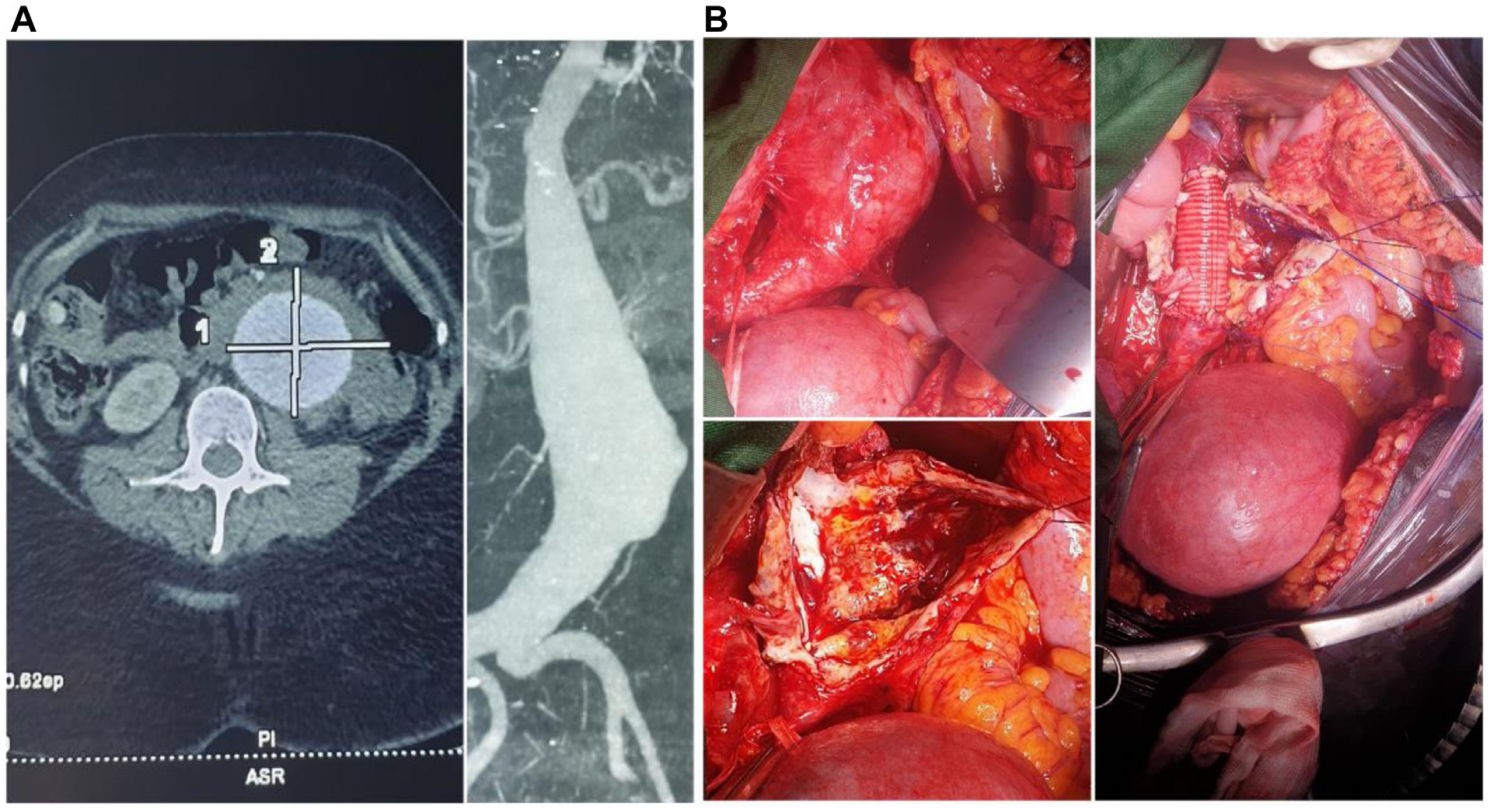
(A) Axial view of angio-computed tomography (CT) scan showing a large abdominal aortic aneurysm (AAA) (left) and two-dimensional MPR reconstruction (right). (B) Intraoperative view of the AAA (upper left corner) before “mise-à-plat” (lower left corner) and interposition of a 22-mm Dacron graft (right).
